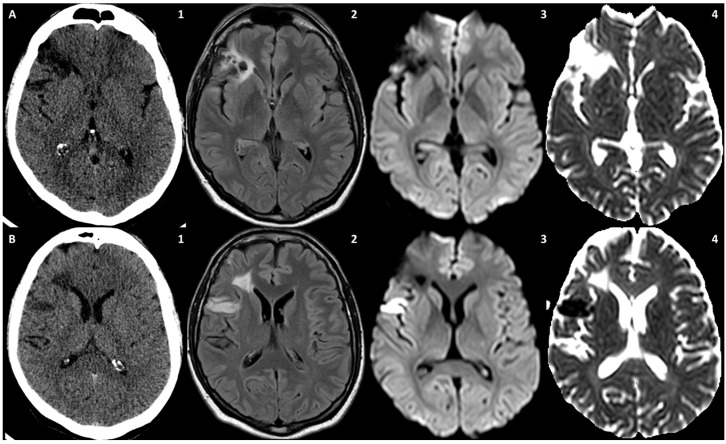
NCCT (24 h before symptom onset) (A1 and B1) and brain MRI (72 h from symptom onset) (A2–A4,B2–B4). (A1) and (B1) are the NCCT axial slices showing the two hypodense lesions in the right MCA territory. (B1) and (B2) are the corresponding T2-FLAIR axial slices. The anterior lesion appears mostly malacic and showed a facilitated diffusion on DWI (A3) and ADC (A4). On the contrary, the posterior lesion showed a restriction on DWI (B3) and ADC (B4), which was more recent.
